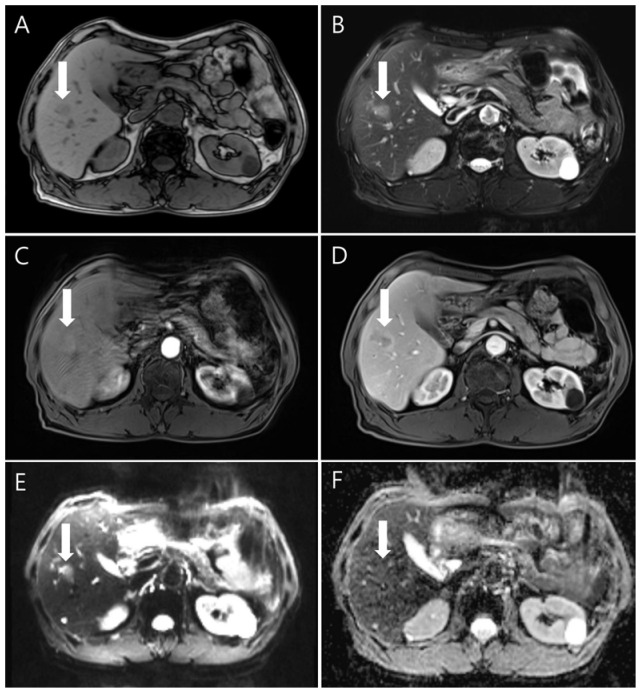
The gadolinium-enhanced dynamic MRI findings showed the nodule (white arrows) with low signal intensity on the unenhanced T1-weighted image (A) and high signal intensity on an unenhanced T2-weighted image (B). In dynamic imaging, the lesion showed slightly high signal intensity in the arterial phase (C) and rim enhancement in the portal venous phase (D). Diffusion-weighted imaging (E) showed indistinct high signal intensity; the apparent diffusion coefficient mapping imaging (F) was isointense.
